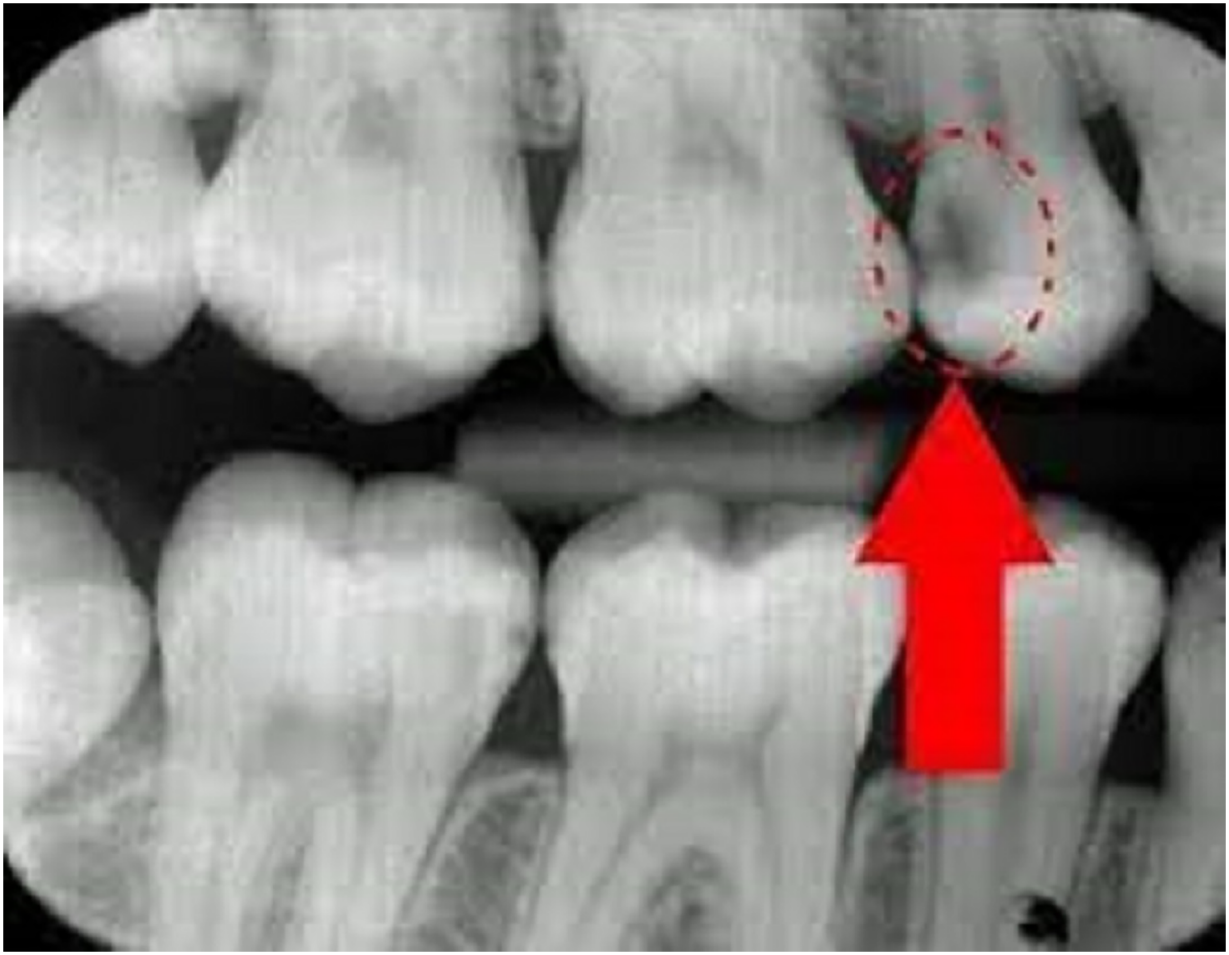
Radiograph explanation of tooth carries and normal.
